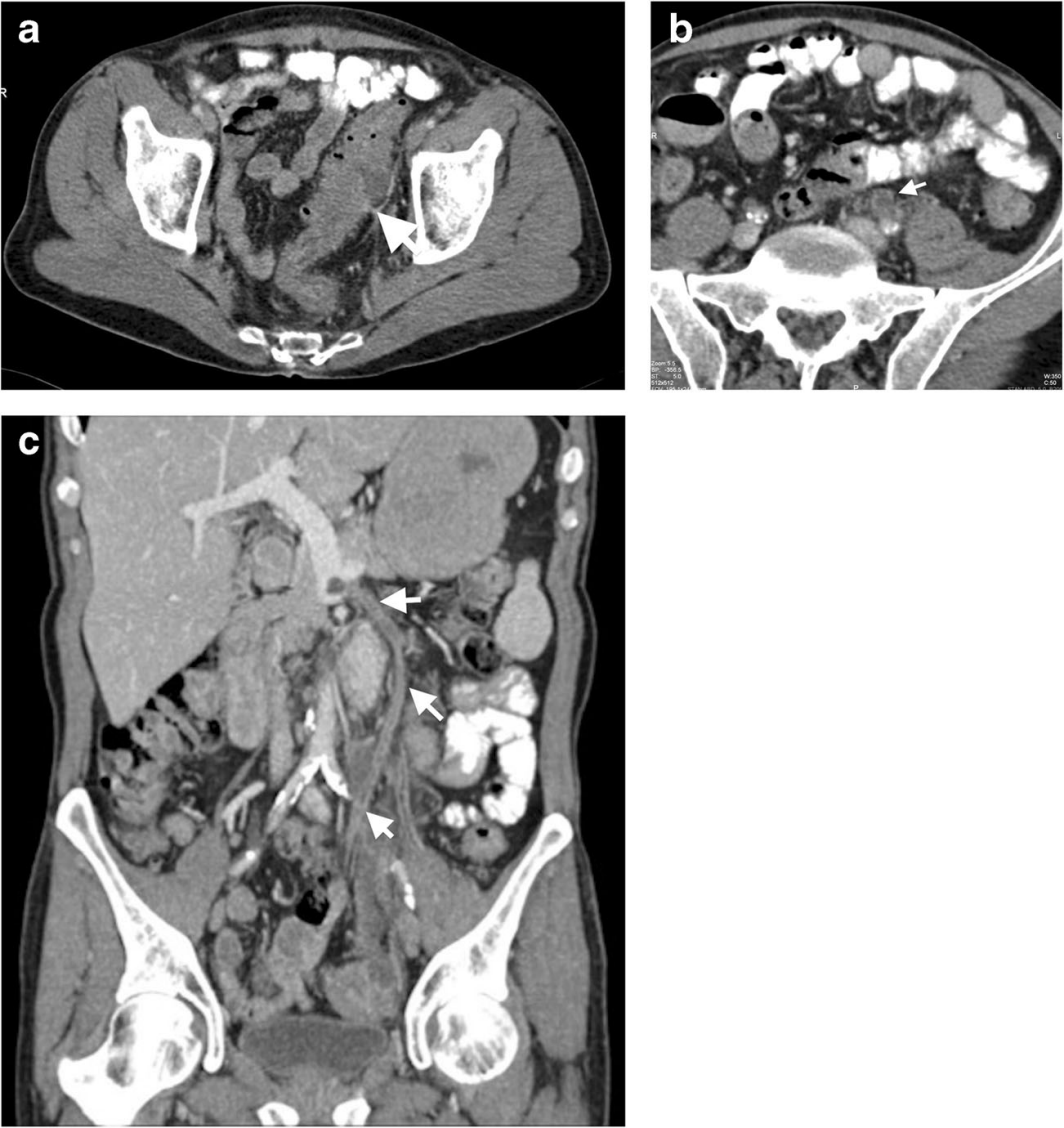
Sixty-year-old male presenting with diverticulitis and IMV thrombosis. a Axial contrast-enhanced CT image demonstrates thickening of sigmoid colon wall (*arrow*) with multiple diverticulum. b Axial and coronal c contrast-enhanced CT images reveal thrombus in the IMV (*arrows*) with adjacent fat stranding representing thrombophlebitis
